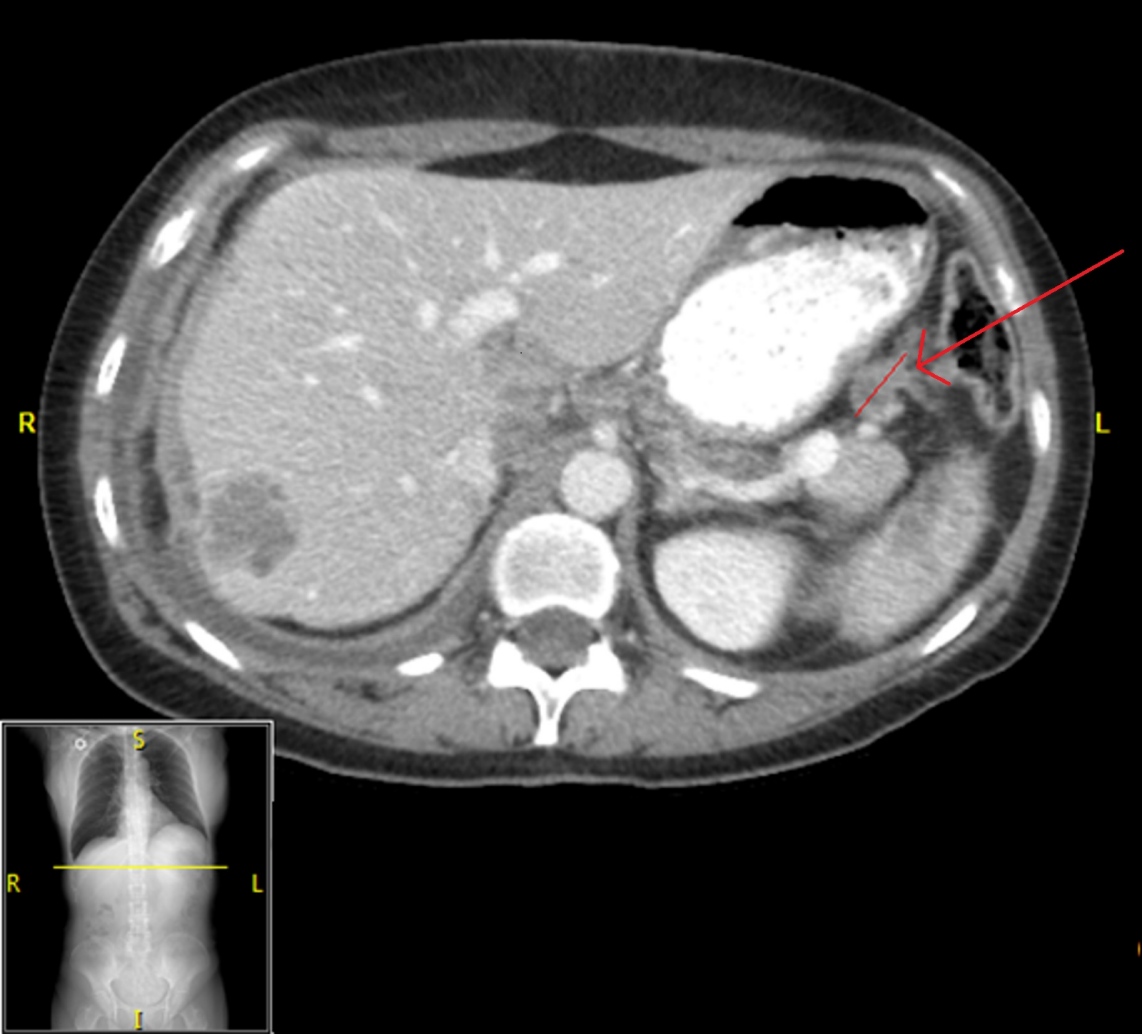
Abdominal CT of Patient 2 after cycle six of evofosfamide, showing an increase in size of target lesion to 2.3 cm in maximal dimension, with CA-125 6185 U/ml
